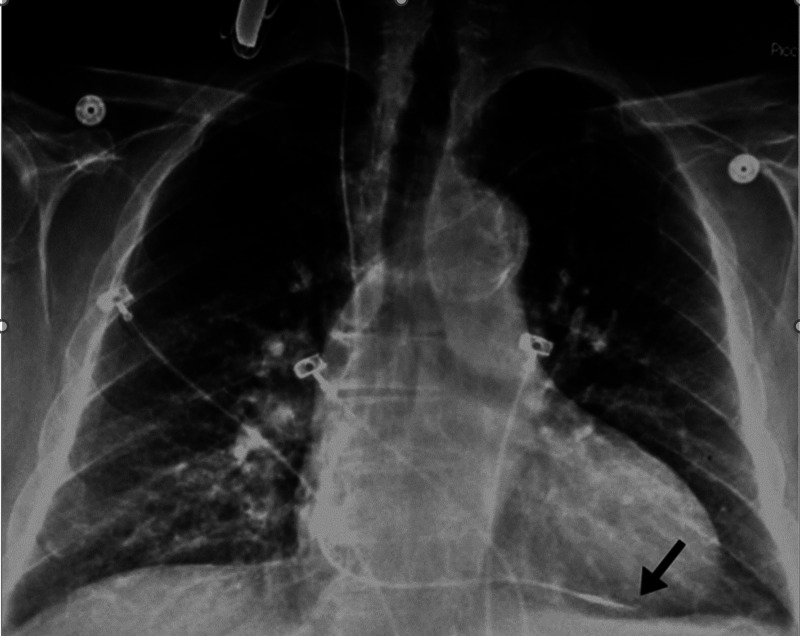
CXR confirming the placement of the transvenous pacemaker tip in the right ventricle (arrow) The figure was used with the written permission of publisher Springer Nature. Permissions were needed, as this CXR was published by Springer Nature. CXR: chest X-ray
